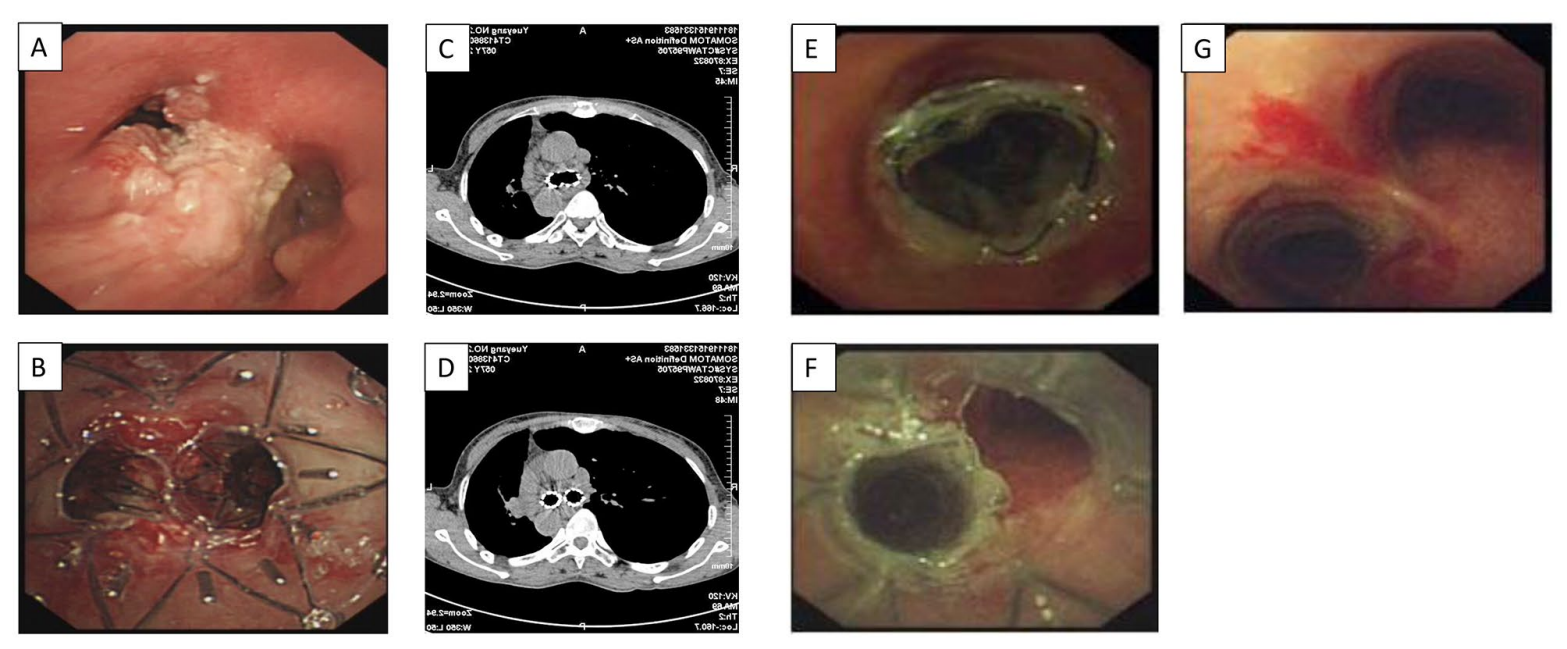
Images of central lung cancer-associated airway obstruction and its treatment with a Y-shape Sigma stent plus I^125^brachytherapy. Panel A: Image of airway obstruction by a malignant tumor located at carina of trachea. Panel B: Image of Y-shape Sigma stent, which was installed at trachea as well as left and right main bronchi following debulking resection of the lung cancer at carina of trachea. Panel C and D: Follow-up images of chest CT after one week of the stent installation. Panel E and F: Images under rigid bronchoscopy after one month of installment of the stent loaded with I^125^. Panel G: Image of carina as well as left and right main bronchi under rigid bronchoscopy after removal of stent at 6 months follow-up
